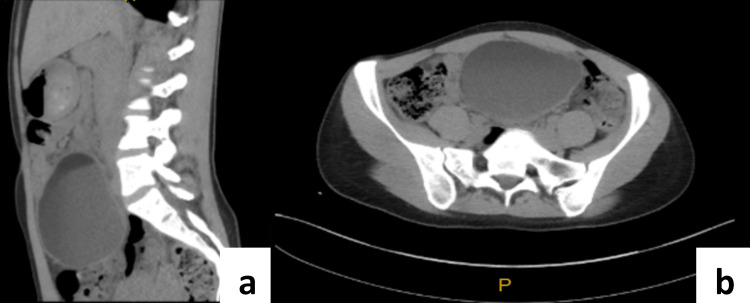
CT images (a: sagittal, b: axial) showing a large central abdominal mass that appears cystic with a fatty component anterosuperiorly and shows no enhancement, most likely a large left ovarian dermoid cyst.
